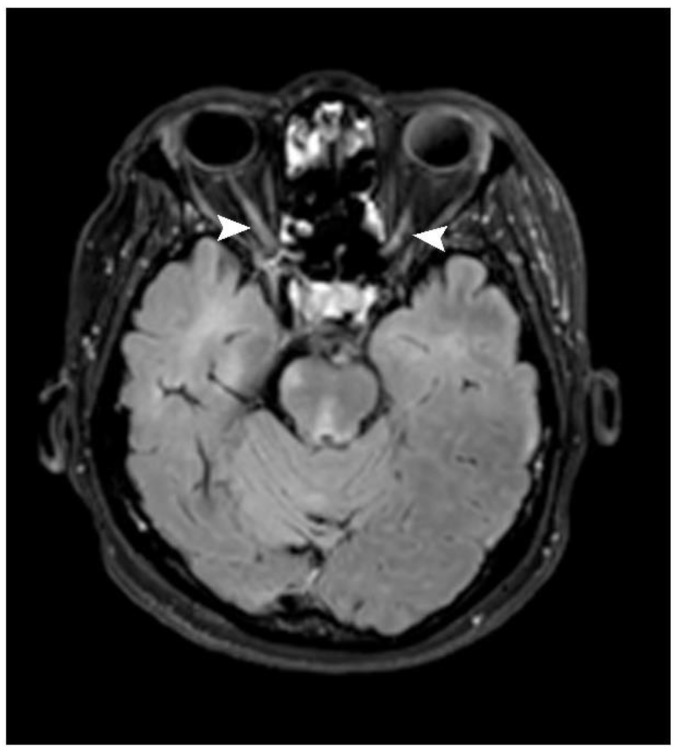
Axial post-contrast FLAIR image shows patchy enhancement at posterior halves of bilateral optic nerves (arrowhead) in keeping with bilateral optic neuritis.
